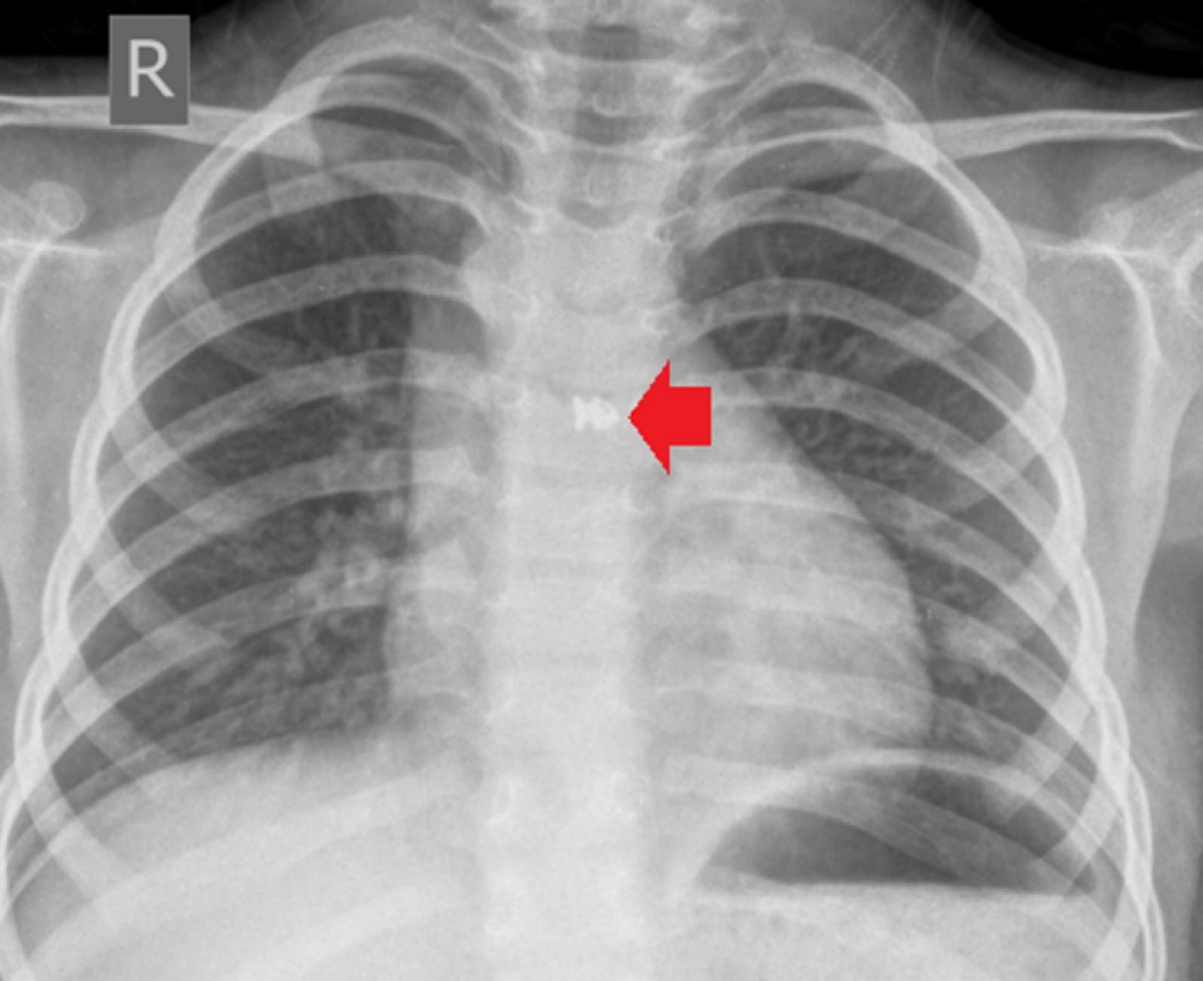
Post-procedure Chest X-ray (CXR): The post-procedure CXR shows the successful deployment of a coil (indicated by the red arrow) used to close the aortopulmonary window (APW). The coil is well-positioned in the area of the former communication between the ascending aorta and pulmonary artery
